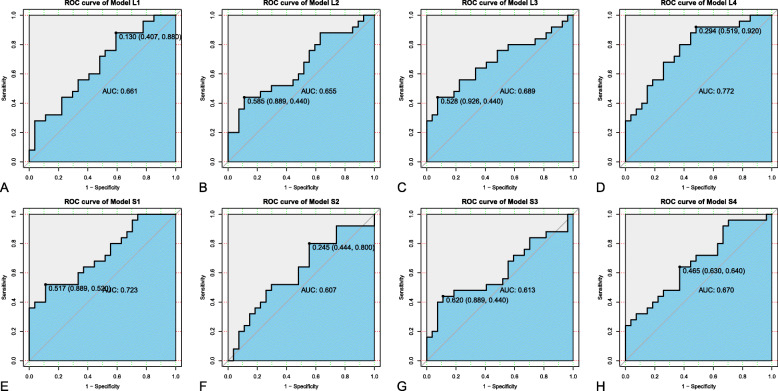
The receiver operating characteristic (ROC) curve plots of the eight prediction of disease progression MRI-based (PDPM) models in the training (A, B, C, D) and validation cohort (E, F, G, H)
